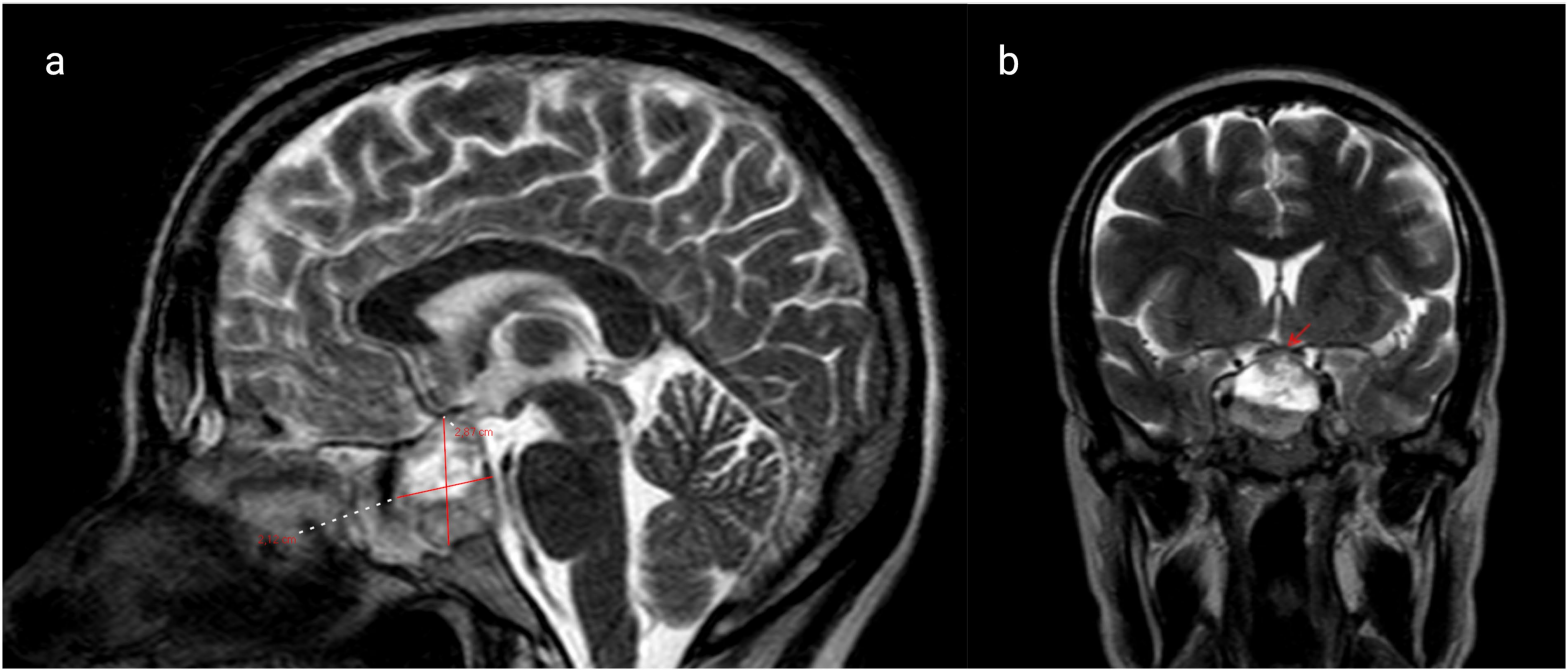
(a) Brain magnetic resonance imaging (MRI) reveals a hemorrhagic macroprolactinoma with dimensions of 28.7 × 21.2 mm (marked with red lines) and (b) coronal T1 post-contrast MRI shows macroprolactinoma impinging on the right cavernous sinus (marked with red arrow).
